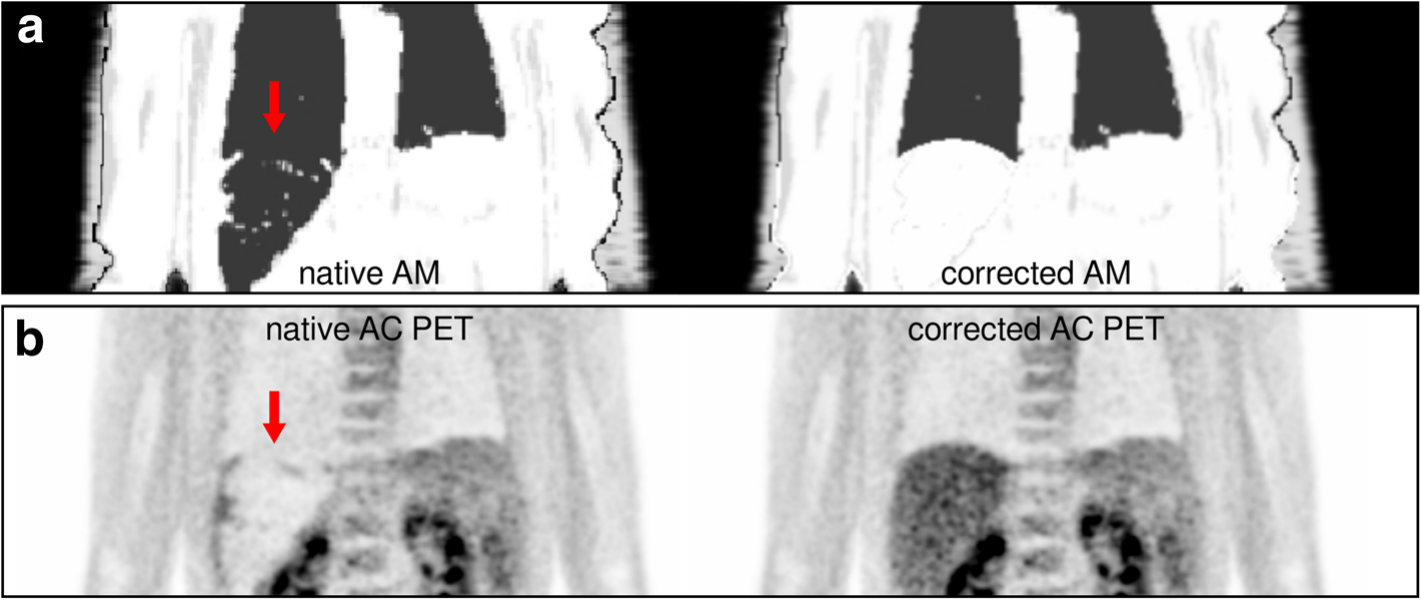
Coronal slice of the MRI-derived attenuation maps (AM; a) and attenuation-corrected [^18^F]FDG PET images (AC PET; b) demonstrating misidentification of liver tissue as lungs and artificially suppressed tracer uptake in the liver (arrows). Native data are shown on the left side while manually-corrected attenuation map and resulting PET data are shown on the right side
